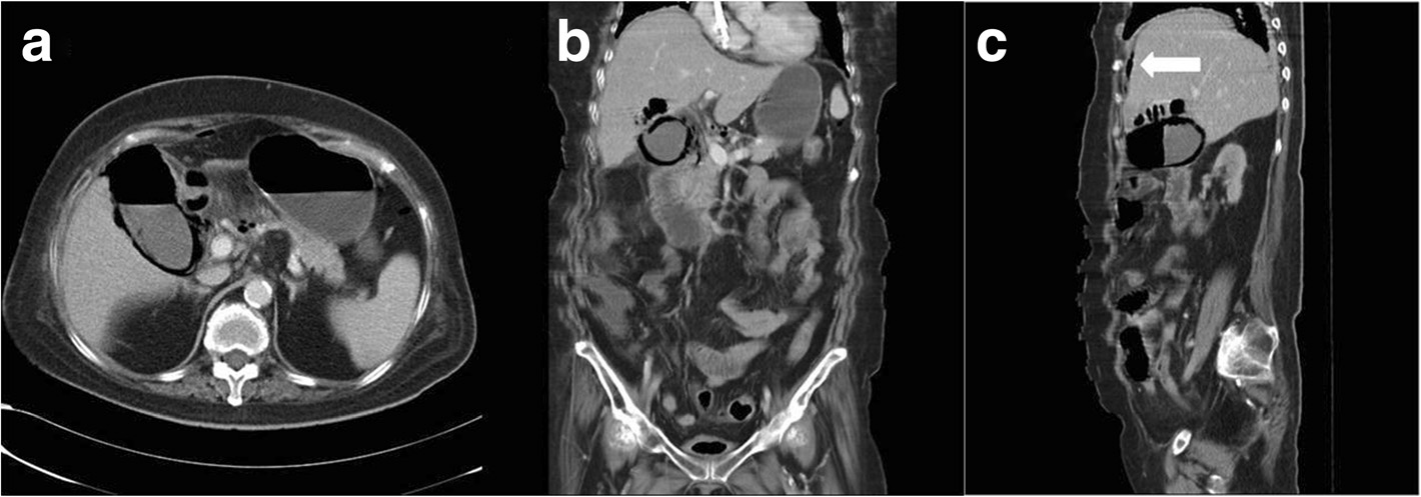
a Computed tomography revealed air encircled the gall-bladder lumen as well as intramural and pericholecystic air pockets, with findings pathognomonic for emphysematous cholecystitis. b Liver parenchymal destruction by air and partial liver abscess denoted. c Pneumoperitoneum as denoted by white arrow
